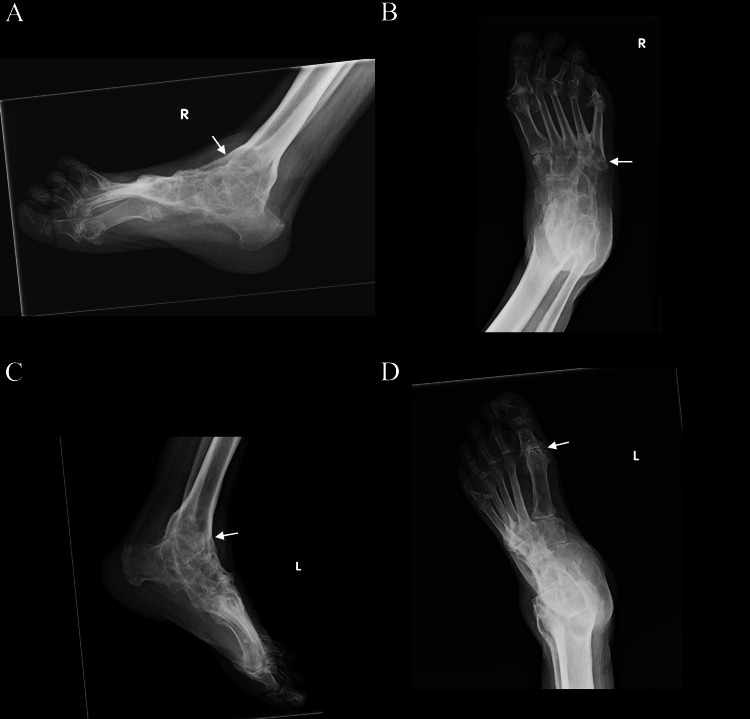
Radiographs of the feet during most recent hospital admission. Lateral views of the right (A) and left (C) foot demonstrate chronic osseous fusion of the right and left ankle joints, posterior subtalar joints, and the transverse tarsal joints. Anteroposterior views of the right (B) and left (D) feet demonstrate erosive changes along the metatarsophalangeal joints, more severe in the right foot compared to the left, and fusion of the second through fifth tarsometatarsal joints of the right foot.
